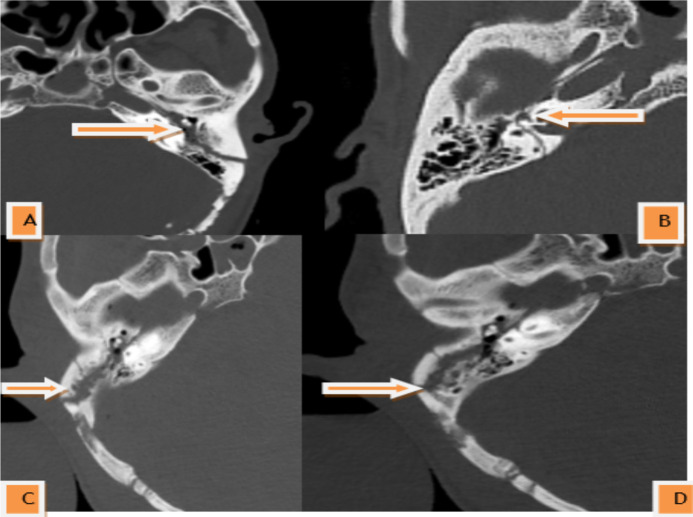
Axial non-contrast bone window CT scan of the head of three different trauma patients showing types of temporal bone fracture based on traditional classification system. Longitudinal fracture(A) transverse fracture (B) and mixed fracture C & D
